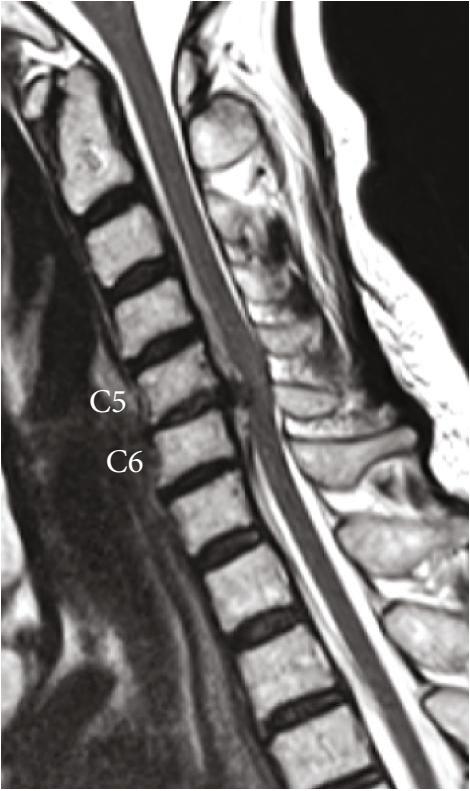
Case 1. Preoperative MRI. T2-weighted sagittal view showing cervical disc herniation at C5-C6 compressing the spinal cord.
